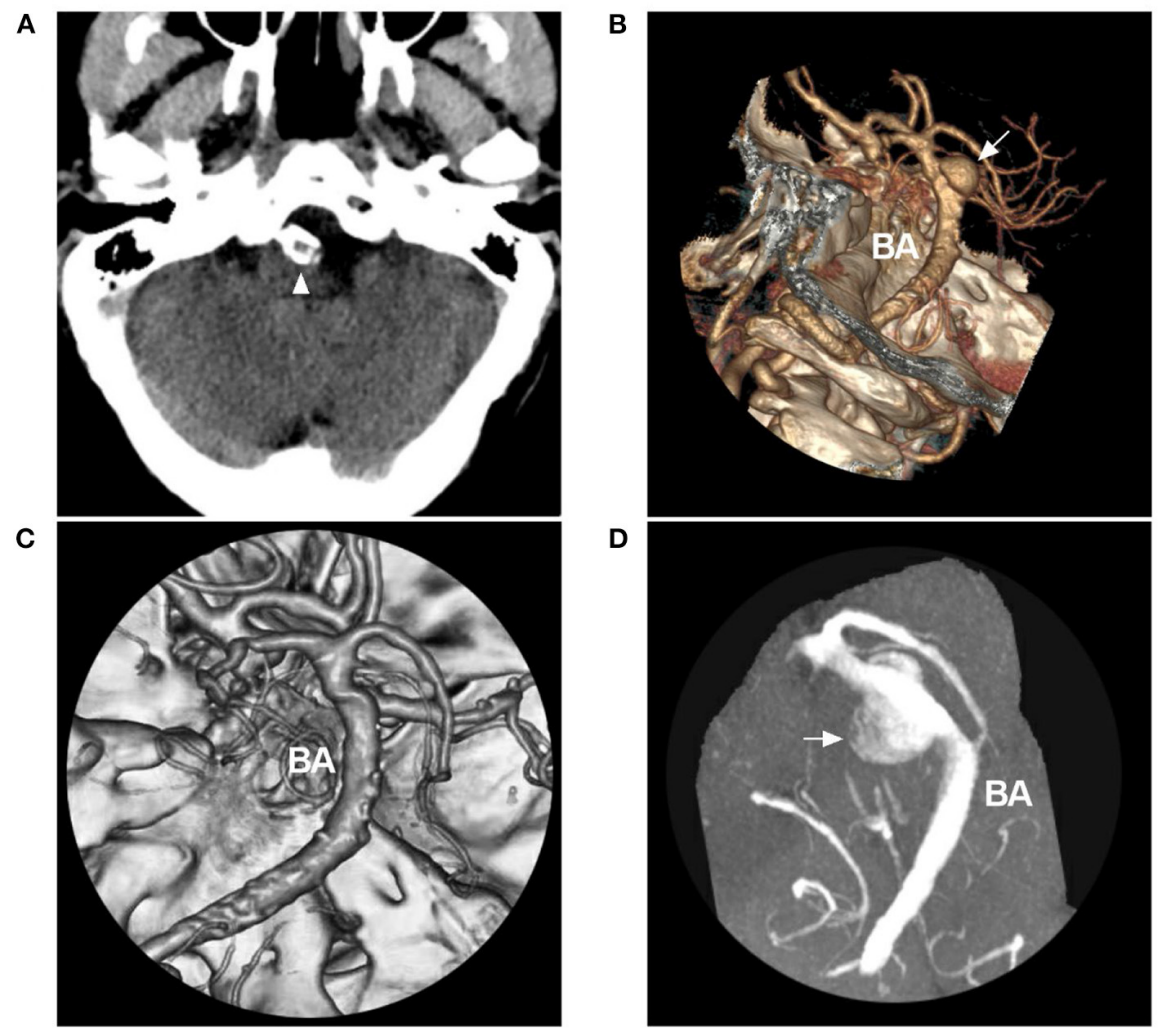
Disguised disappearance of associated aneurysm in VBD. (A) CT showing BA calcification (arrowhead). (B) CTA showing an aneurysm at the BA (arrow) in a VBD. (C) Six-month follow-up CTA suggesting disappearance of the aneurysm. (D) Magnetic resonance angiography showing that the disappearance of the aneurysm was disguised due to thrombosis in the aneurysm. The patient suddenly fell into a coma and died within 1 h after 1 month of the last follow-up CTA. Rupture of the aneurysm was considered. BA, basilar artery; CT, computed tomography; CTA, computed tomography angiography; VBD, vertebrobasilar dolichoectasia.
